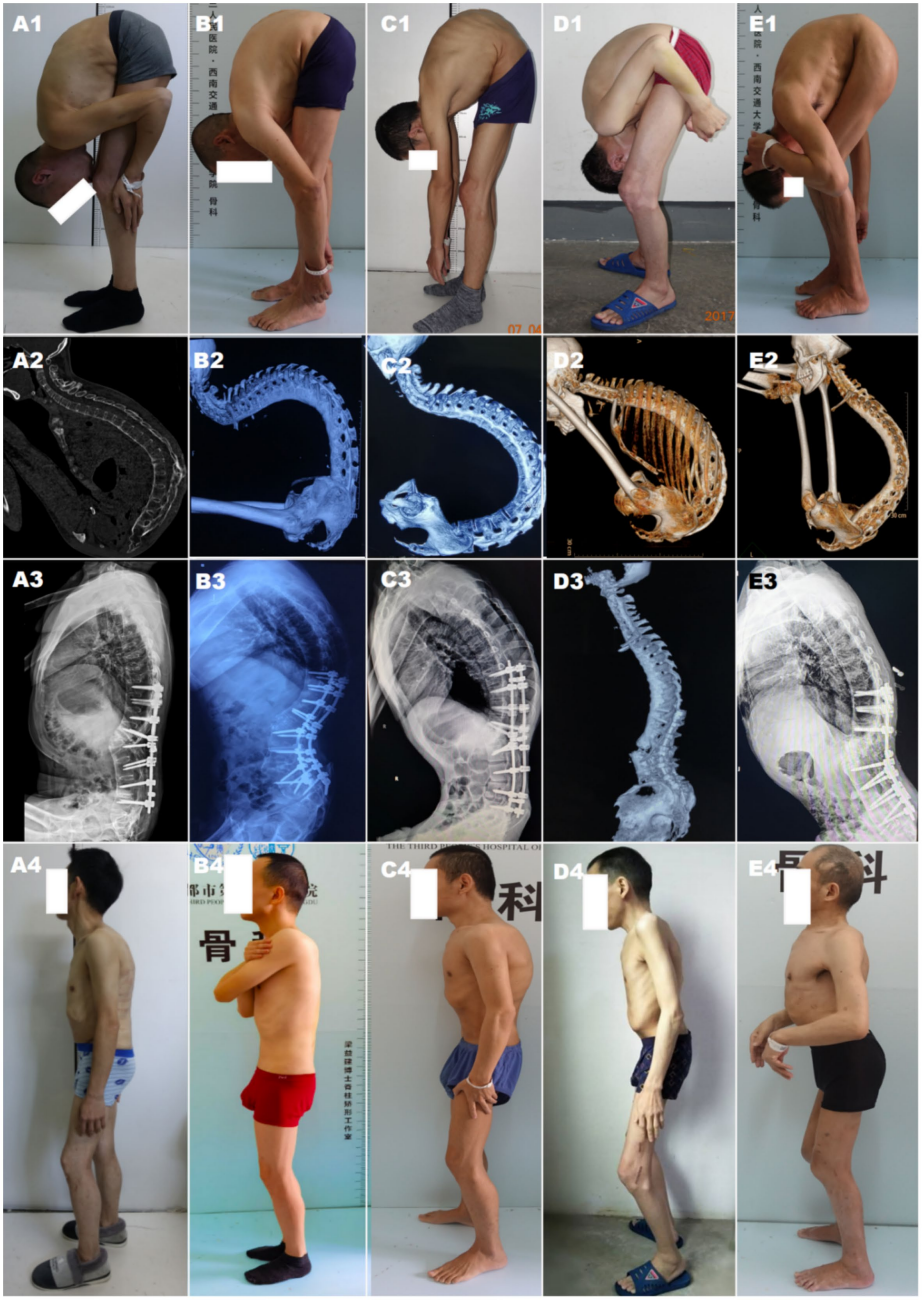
Pre-and postoperative presentation and changes in radiographic spinal level maps. (A–E) represent five patients. (A1–E1) Preoperative standing posture showing thoracolumbar kyphotic deformity; (A2–E2) Preoperative 3D CT reconstruction of the spine; (A3–E3) Postoperative spine alignment shown on X-ray/CT; (A4–E4) Postoperative standing posture changes.
